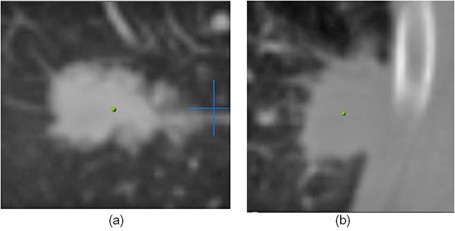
A CT slice image (a) of an island‐type lung tumor, which features the high‐density tumor completely surrounded by lower‐density lung parenchyma. A CT slice image (b) of a lung wall‐seated tumor in which the tumor abuts the chest wall and resides near lung‐adjacent OARs, such as the rib in this image.
